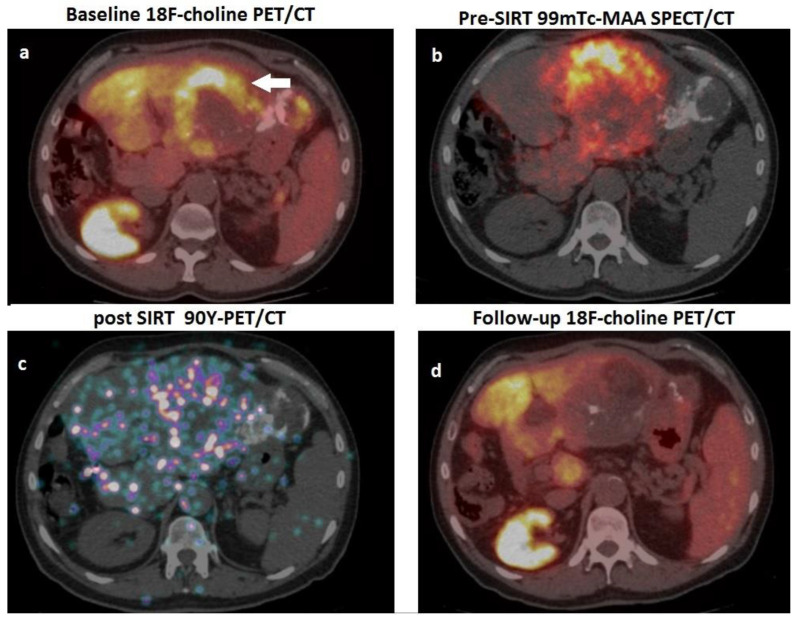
A 62-year-old man, affected by HCV-related cirrhosis, presenting a gross HCC of the left hepatic lobe, previously submitted to surgery (moderately differentiated HCC, pT2), subsequently relapsed and was treated with TACE. One year after TACE, he was referred to our department due to relapse and increased value of AFP (2357 ng/mL) for possible treatment with ^90^Y-microspheres. (a) Pre-treatment PET/CT scan with 18F-choline showed a hemicircular rim of increased tracer uptake (white arrow) within the HCC lesion in the left hepatic lobe (note the hyperdense material in the neighbouring parenchyma due to the previously performed TACE). (b) The patient was submitted to scintigraphy with albumin 99mTc-macroaggregates (i.e., PRE-SIRT), and coregistered SPECT-CT images showed an optimal match with respect to the location of the metabolically active lesion detected by PET/CT; (c) After selective catheterisation of the arterial feeder of the HCC lesion, 1.8 Gbq of 90Y-resin microspheres were administered; PET/CT acquired on 90Y-positron emission showed microspheres’ accumulation within the hepatic lesions; (d) 18F-choline PET/CT performed eight weeks after SIRT showed an almost complete metabolic response, consistent with a dramatic drop in AFP values (i.e., 50 ng/mL).
